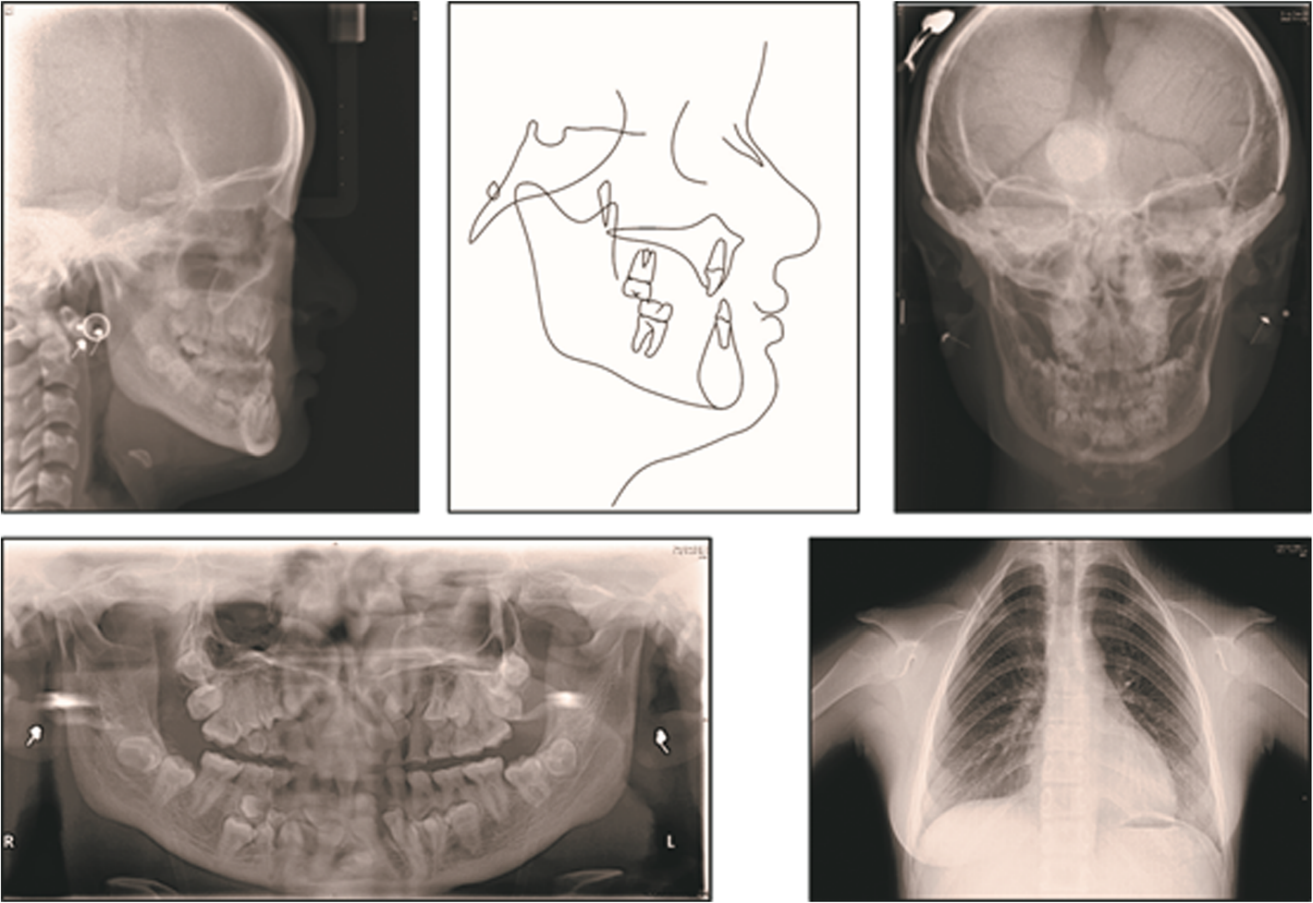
Pretreatment chest radiograph, panoramic radiograph, frontal radiograph, lateral radiograph, and cephalometric tracing
